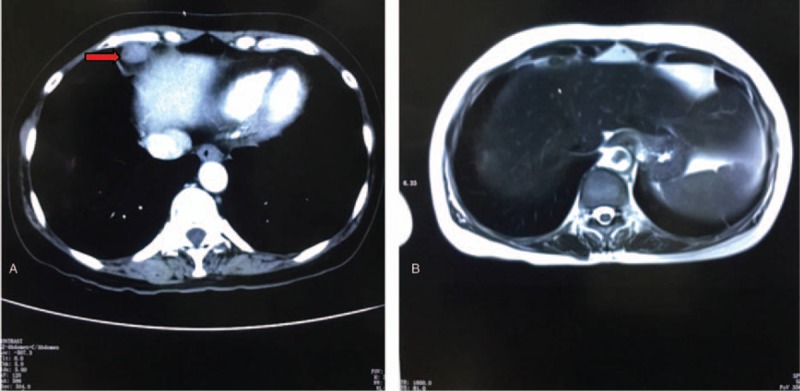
(A) CT image showing liver metastases before and (B) MRI scan showing that the mass on the liver capsule had disappeared after treatment with apatinib and epirubicin. CT = computed tomography, MRI = magnetic resonance imaging.
